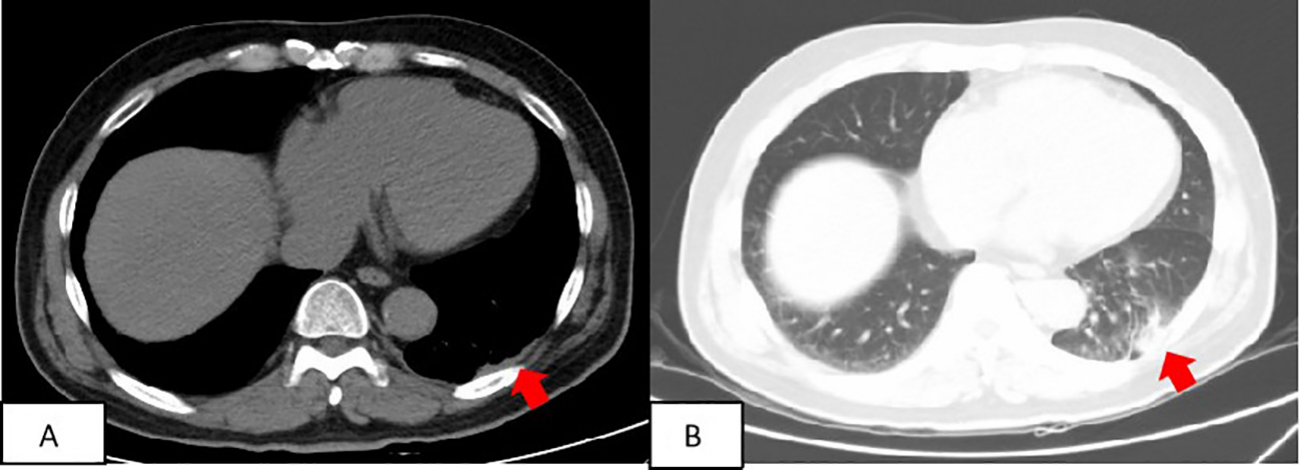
Patient’s follow-up findings: two and a half years after surgery. At the patient’s most recent follow-up, conducted two and a half years after the surgery, a computed tomography (CT) scan revealed a slight thickening of the left pleura (A) and mild chronic inflammation in the lung tissue surrounding the surgical site (B).
